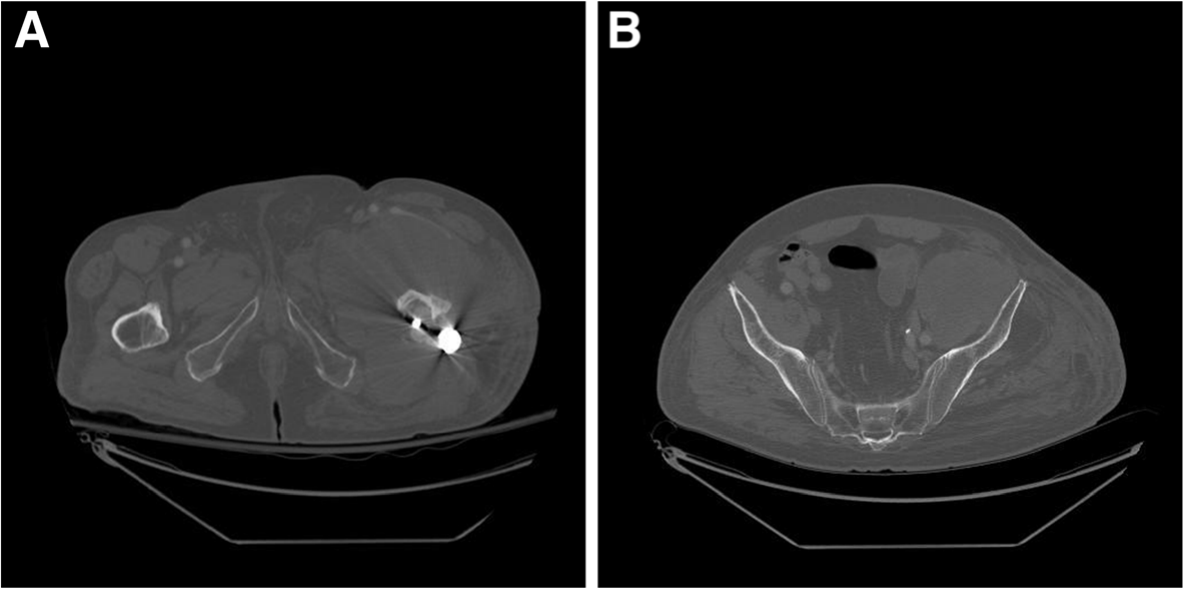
Axial computed tomography of pelvis. A: A dense collection/mass in anterior left thigh with lateral extension at level of the proximal femur. B: Additional cuts revealing that the collection/mass extends to the pelvis.
